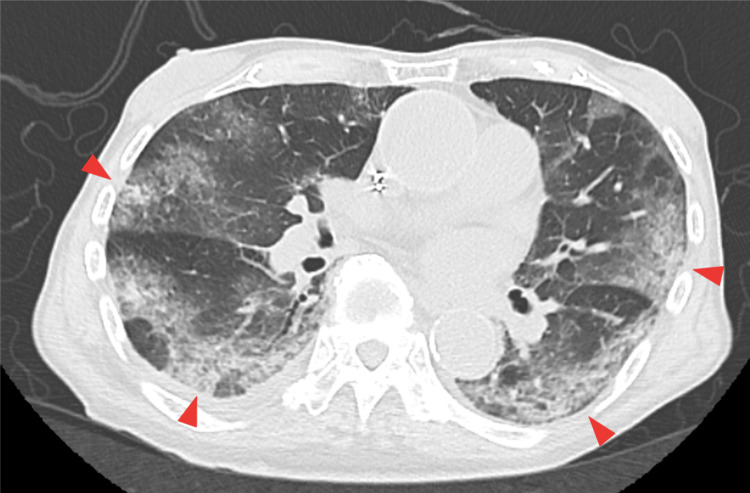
Bilateral ground-glass opacities and consolidations on admission chest CT. Non-contrast axial chest CT on the day of admission showing bilateral ground-glass opacities and peripheral consolidations (arrowheads), consistent with COVID-19 pneumonia. These findings correspond to a CO-RADS score of 5, indicating a very high level of suspicion for COVID-19, consistent with the positive antigen test result. The lesions are predominantly distributed in the posterior and peripheral regions of both lungs, demonstrating a typical radiological pattern of SARS-CoV-2 infection. These findings supported the clinical diagnosis and guided the initiation of antiviral and corticosteroid therapy. CO-RADS: COVID-19 Reporting and Data System
